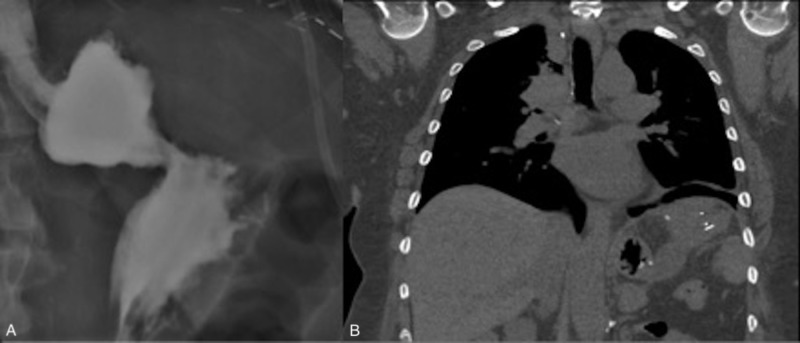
(A) Fluoroscopic upper GI series with oral contrast on post-operative day three shows no leakage of contrast outside of the stomach; (B) CT scan on post-operative day five shows no evidence of communication between the stomach and the pleural space GI: gastrointestinal, CT: computed tomography
